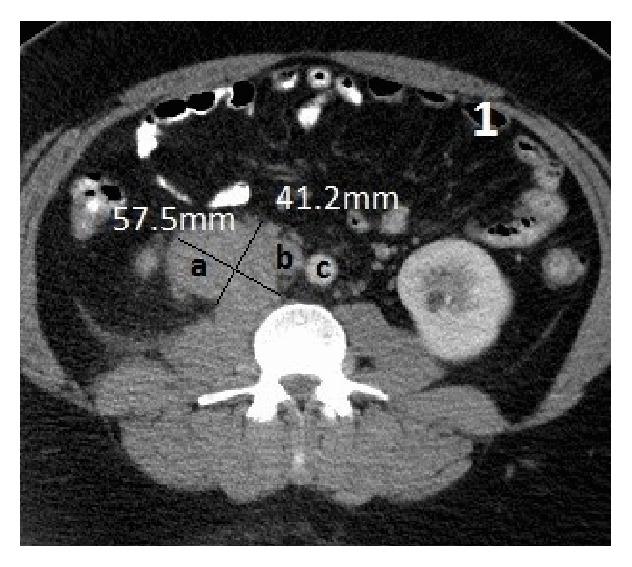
Abdomen CT axial view: (a) inferior vena cava (IVC) varix measuring 57.5 mm × 41.2 mm, (b) IVC, and (c) aorta.
